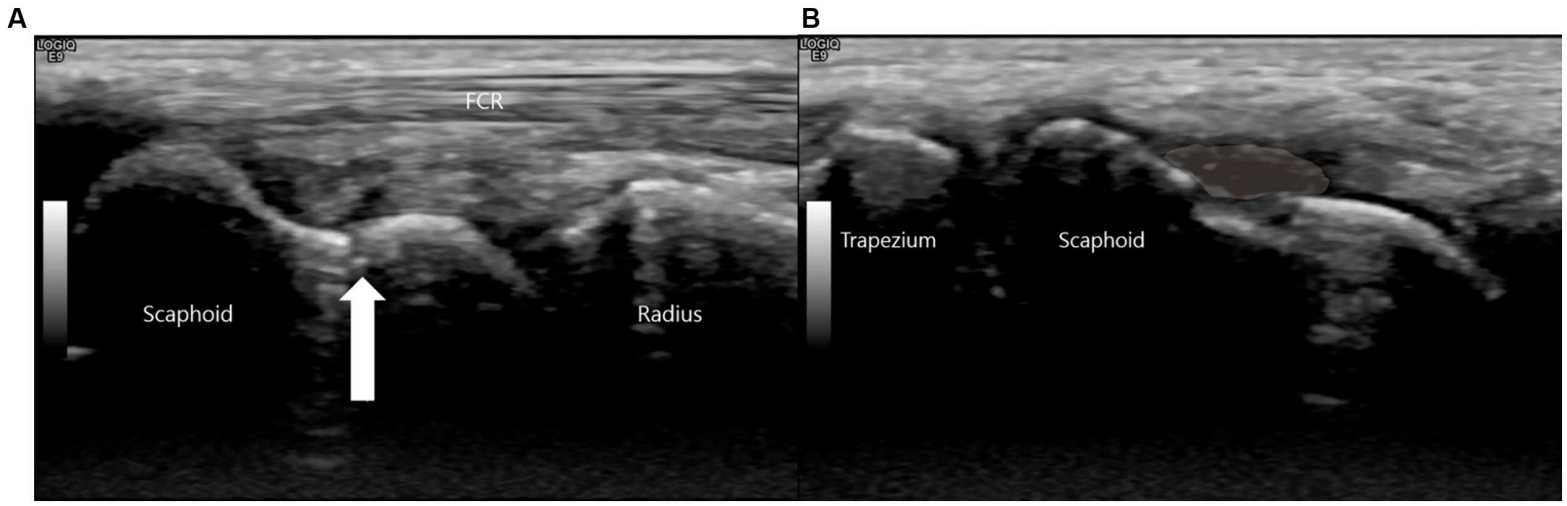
This is a high-resolution ultrasound image of the scaphoid waist. (A) The white arrow indicates the fracture site. (B) A hypoechoic collection consistent with a hematoma (shaded area) is noted superficial to the cortical breach.
